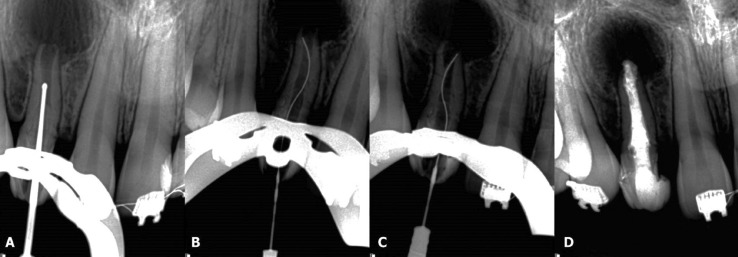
A. An ultrasonic tip was carefully used to remove the invaginated tissue. B and C. Radiographs showing the XP-endo Finisher instrument touching the canal walls. D. Final radiograph presenting the root canal filling of type II DI.
